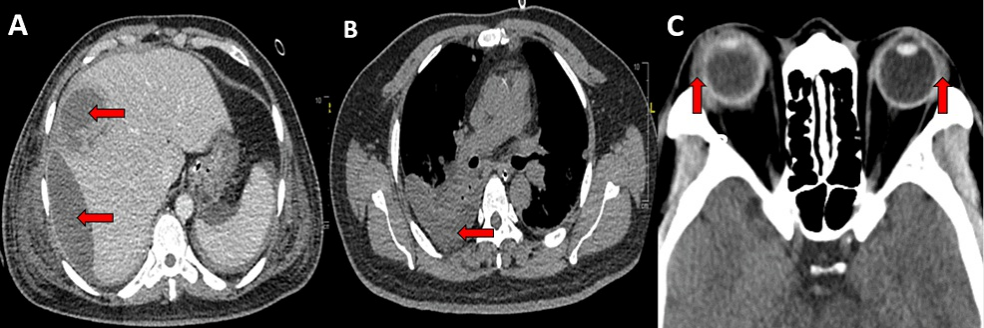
Contrast-enhanced computed tomography (CECT) scans A) CECT liver 4-phase shows irregular multiloculated hypodense collection at segment V/VIII suggestive of ruptured multiloculated liver abscess. B) CECT thorax shows loculated right pleural effusion with enhancing pleural outline representing empyema. C) CECT orbits shows irregular and enhancing scleral thickening in both globes with periorbital soft tissue thickening suggestive of panophthalmitis.
